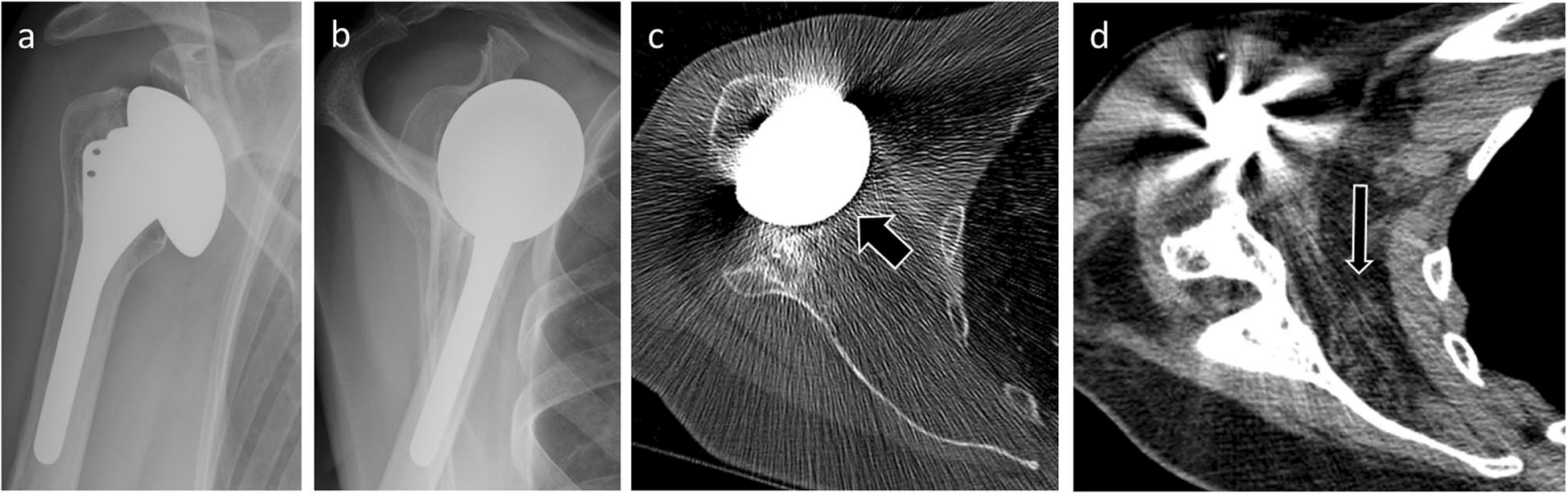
Anteroposterior (a) and scapular Y (b) radiographs in a 52-year-old woman with total shoulder arthroplasty and anterior instability show an anterior translation of the humeral head. c Axial CT image confirms this anterior translation (thick arrow). d Axial CT image with smooth reconstruction filter shows fatty degeneration of the subscapularis muscle (thin arrow). All these elements lead to suspicion of subscapularis tendon tear and this tear was confirmed during surgery
